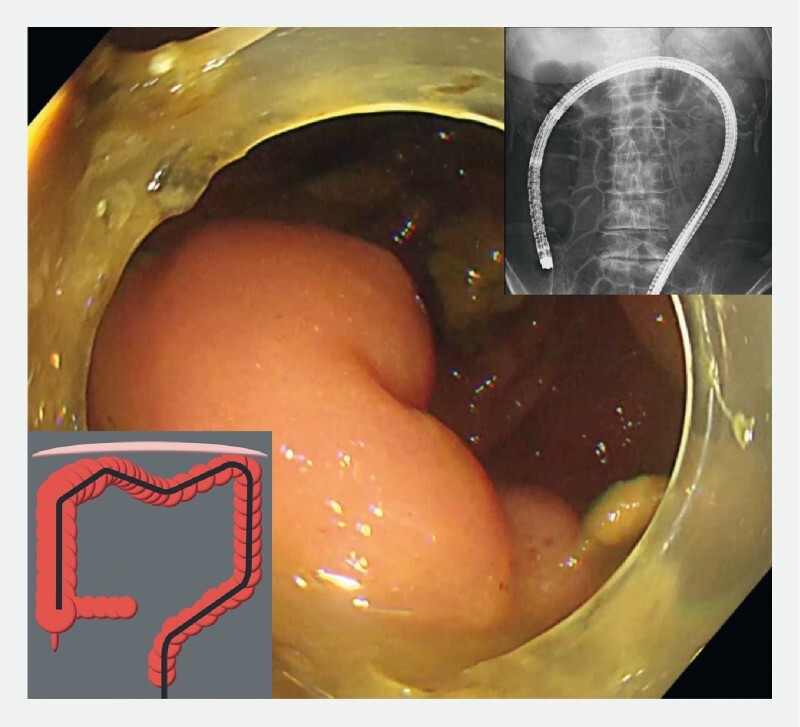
The endoscope was inserted to the ileocecal valve, and hernia reduction was confirmed by X-ray fluoroscopy.
